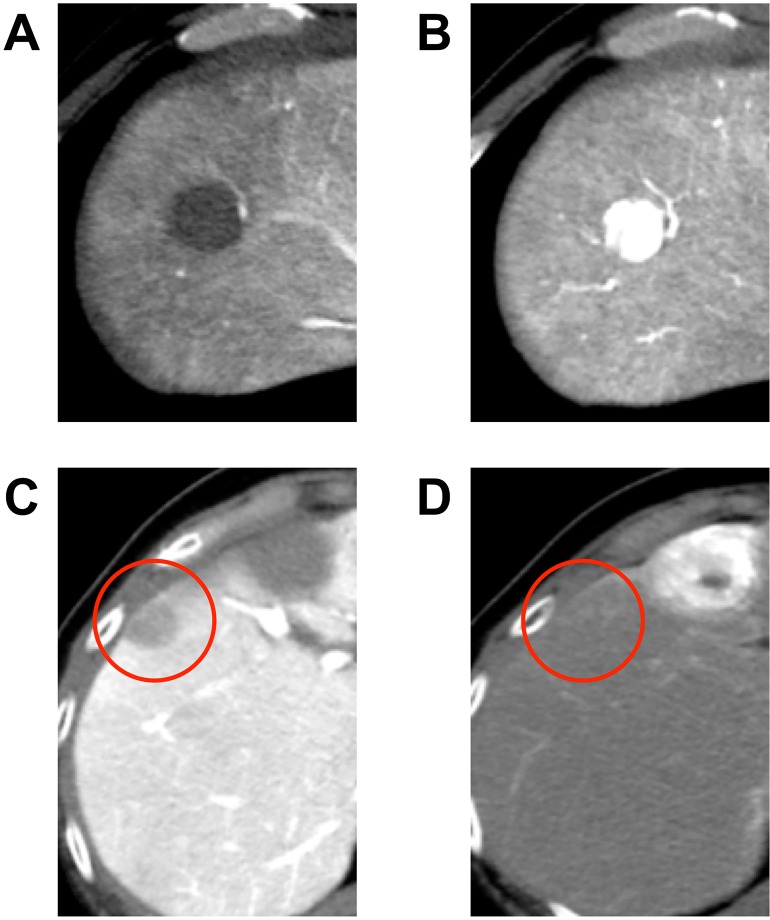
A typical nodule of hypervascular hepatocellular carcinoma (HCC) (A: CTAP, B: CTHA) and a hypovascular nodule (red circle, C: CTAP, D: CTHA). CTAP, computed tomography during arterial portography; CTHA, computed tomography during hepatic arteriography.
